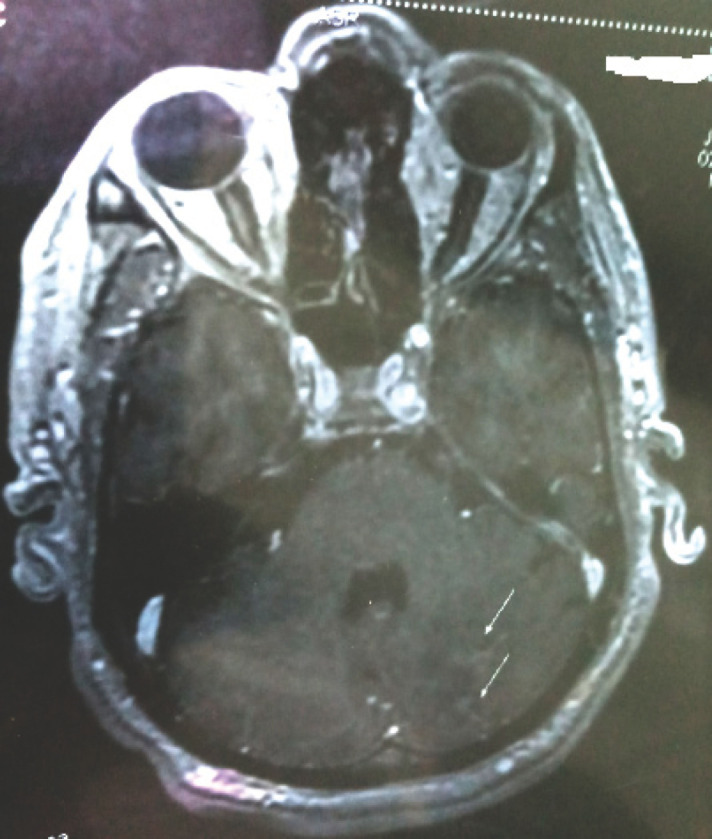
Micro-abcès sous-tentoriels à l’imagerie par résonnance magnétique (IRM) Subtentorial micro-abscesses on magnetic resonance imaging (MRI)
